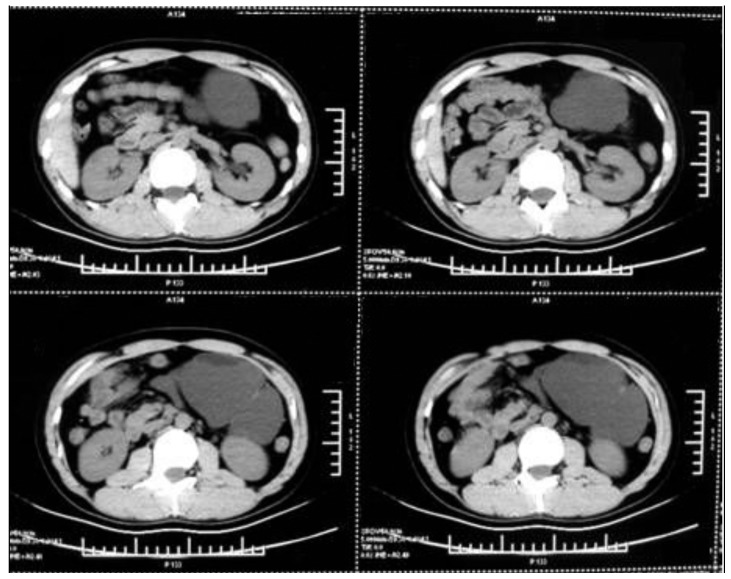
Computed tomography scan indicating a huge cystic mass in the midabdomen, with an estimated maximum diameter of >15 cm.
